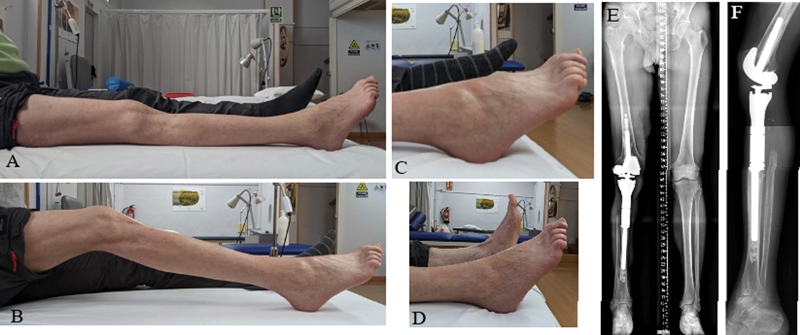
Final postoperative images. A) Knee extension , B) Knee flexion C) Full plantar flexion D) Dorsal flexion deficit (−40°). E and F) Postoperative full-length and lateral x-ray images show a correct implantation of the endoprosthetic replacement.
